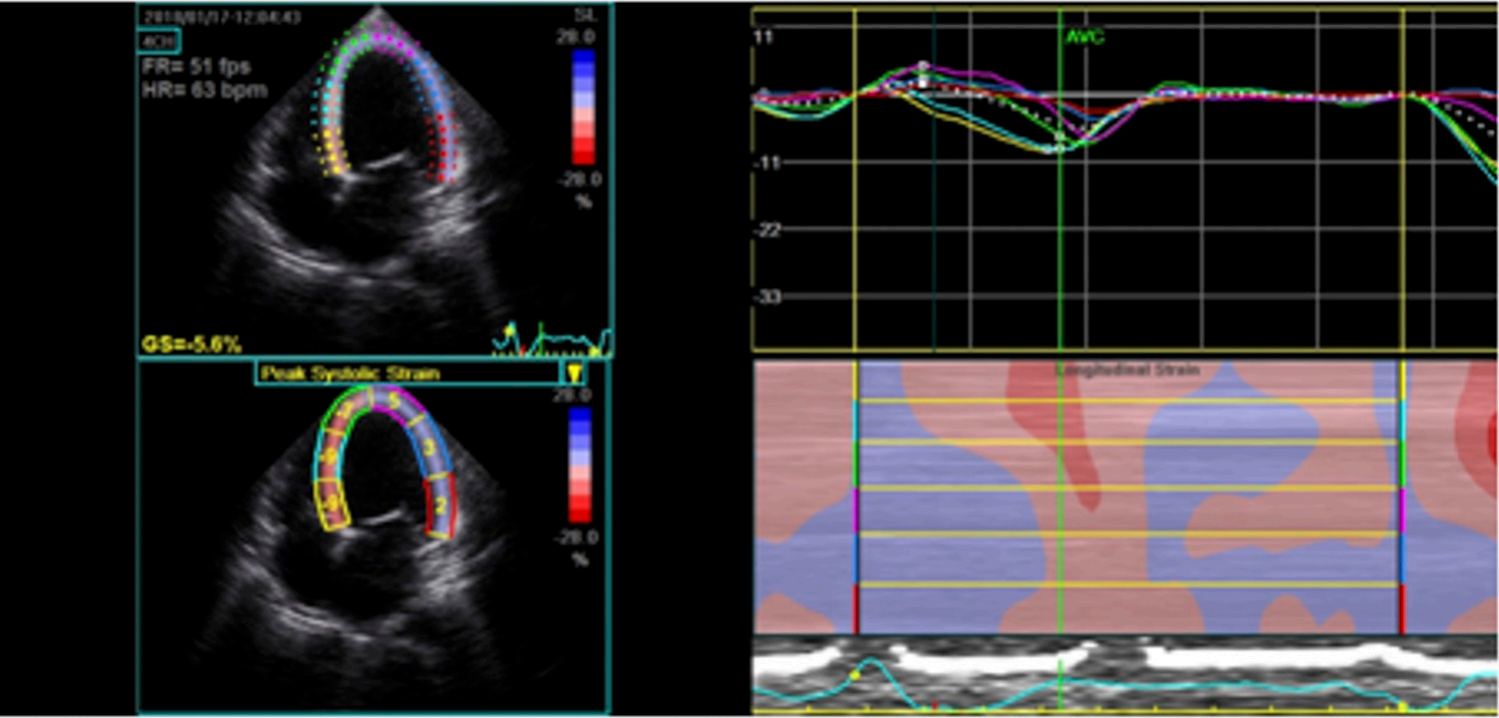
Peak systolic longitudinal strain of the left ventricle obtained at apical 4-chamber view. Parametric (color-coded) display of end-systolic strain (left upper panel); segmental peak systolic strain values are indicated (left lower panel); strain-time curves: colored curves displaying segmental strain (right upper panel); M-mode representation of peak systolic strain (right lower panel).
